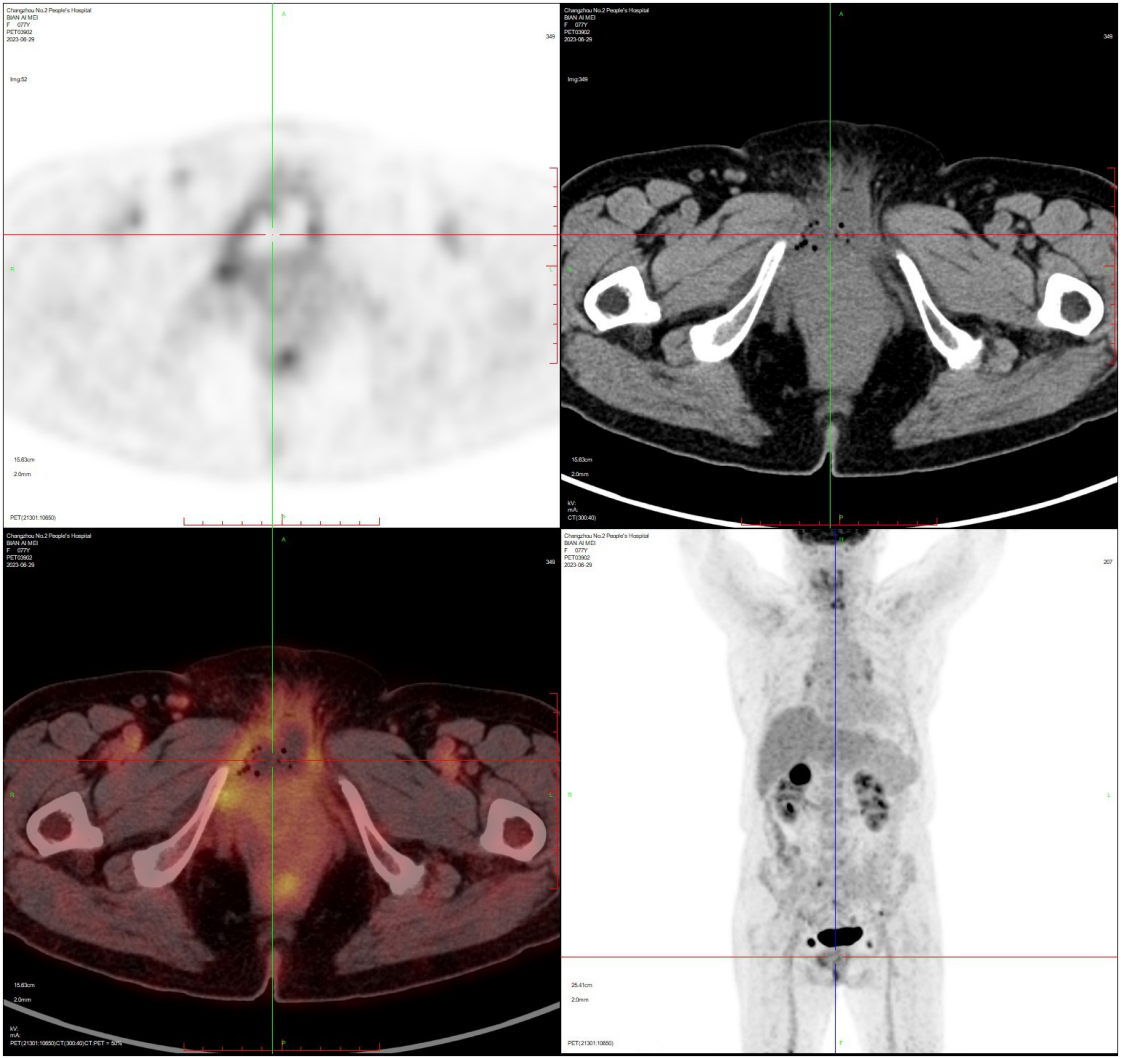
PET-CT after vulvar lymphoma surgery.
